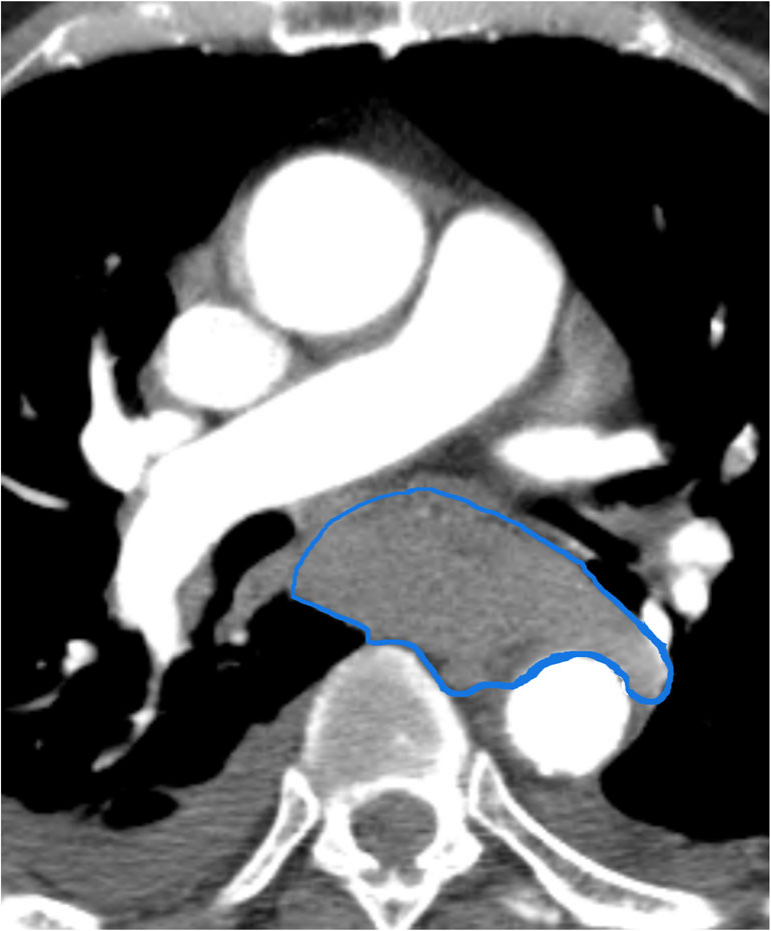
Intramural Hematoma. CT angiogram of the chest in soft tissue window shows a markedly thickened oesophageal wall that is slightly higher in density than soft tissue. The oesophageal wall partly surrounds the aorta. This patient was found to have a large intramural hematoma
